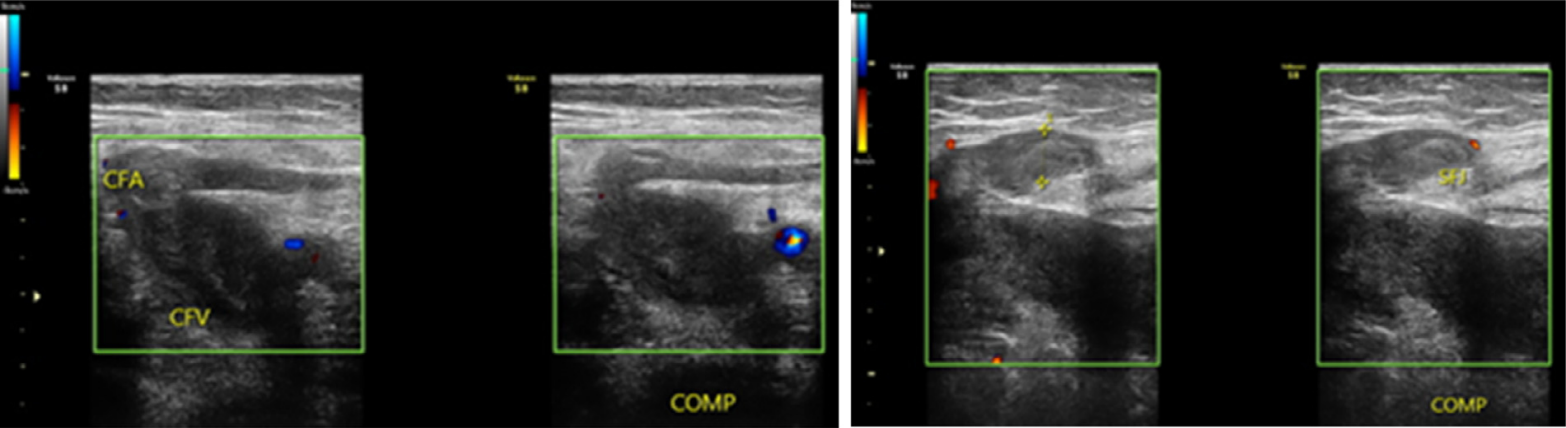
Left lower extremity Doppler ultrasound examination shows no flow in a noncollapsible vein with thrombus in the lumen of the left greater saphenous vein in section with the left common femoral vein (left) and left superficial femoral vein (right).
